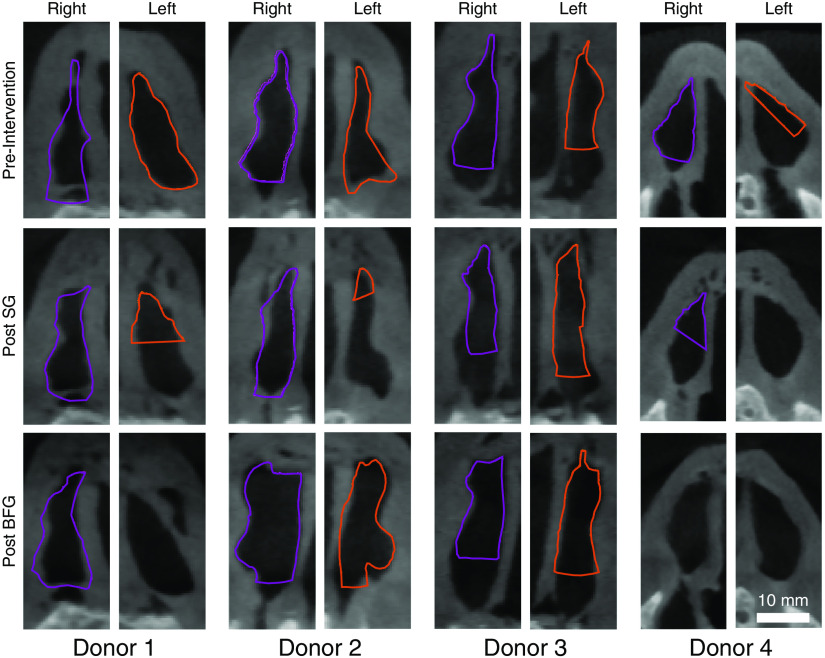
Contours of the INV determined from aOCT scans (purple, right INV; orange, left INV) overlaid on corresponding CT coronal images. SG, spreader graft; BFG, butterfly graft.
